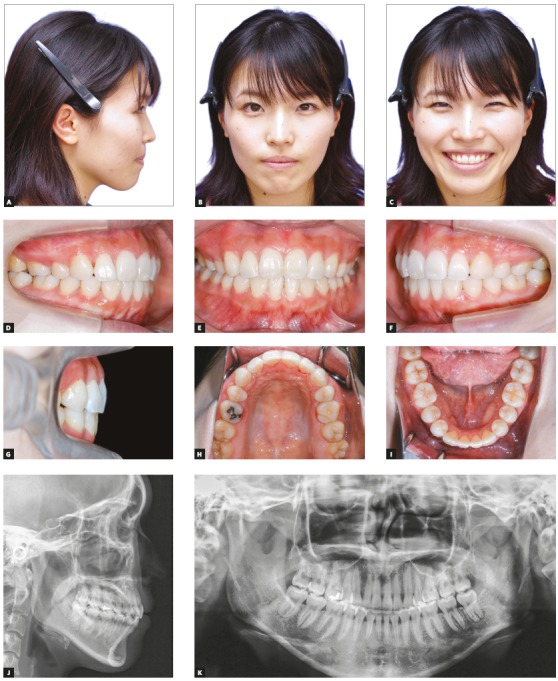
Posttreatment records (at 24 years and 2 months of age): A-I) extraoral and intraoral photographs; J) lateral cephalometric radiograph; K) panoramic radiograph.
